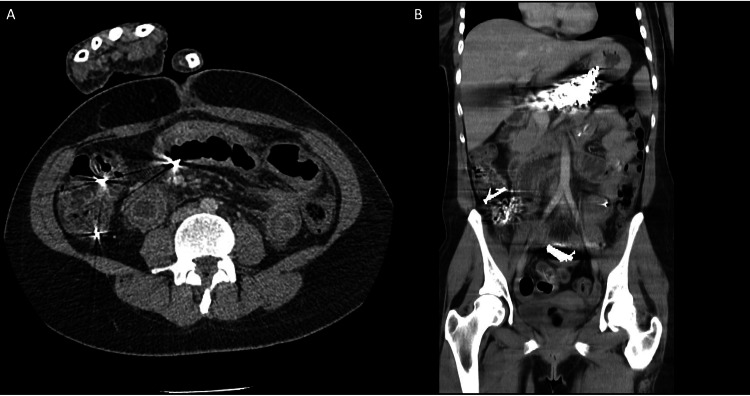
Abdominal CT scan demonstrating extensive ingestion of metallic foreign bodies. (A) Axial view showing numerous metallic objects within the gastric lumen and small bowel loops, with evident segmental wall thickening of the small intestine and adjacent fat stranding.
